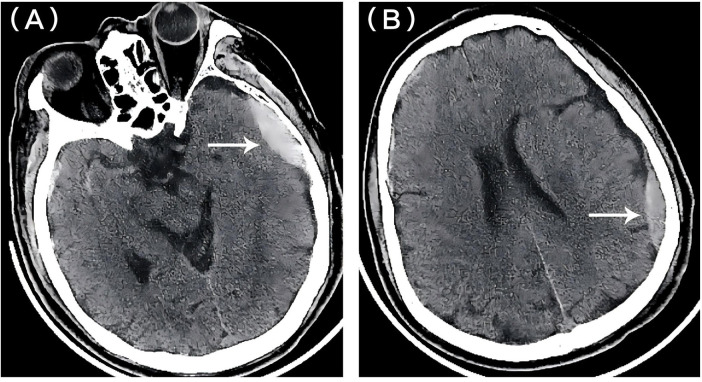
Initial noncontrast head CT demonstrating a left-sided epidural hematoma (EDH). (A) Axial CT image at the basal skull level (lower slice) showing the epidural hematoma (arrow) with a maximal thickness of approximately 1.2 cm. (B) Axial CT image at the level of the lateral ventricles (higher slice) revealing the epidural hematoma (arrow), which appears thinner. There is no significant mass effect or midline shift in either slice.
